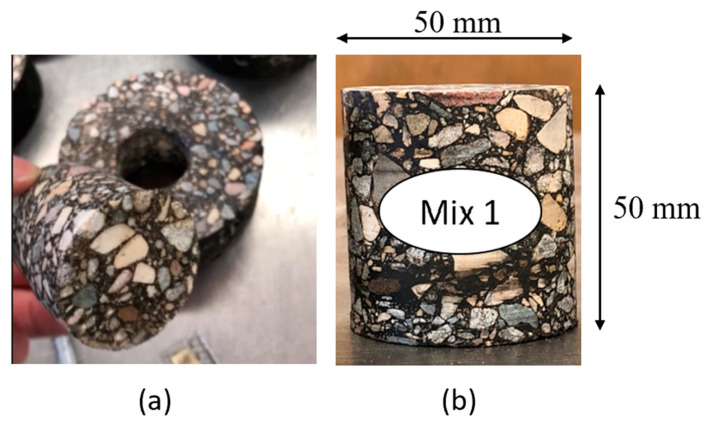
AC sample for X-ray CT scan: (a) sample extraction; (b) the dimensions of the extracted sample.
